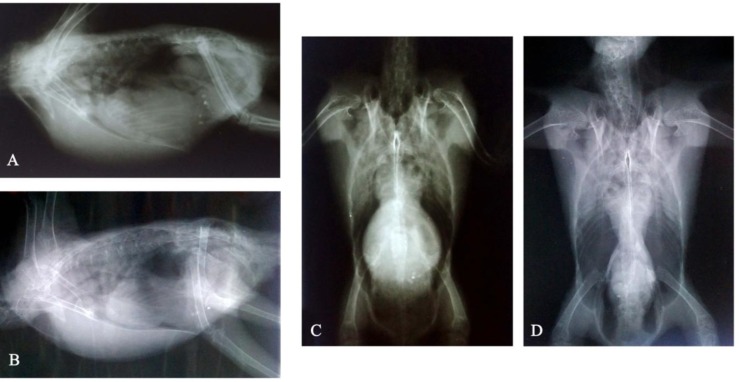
Lateral and ventrodorsal radiograph before (A and C, respectively) and after (B and D, respectively) treatment. Hepatomegaly and renal enlargement was seen in A and C. In B and C the kidney and liver size are within normal limit
